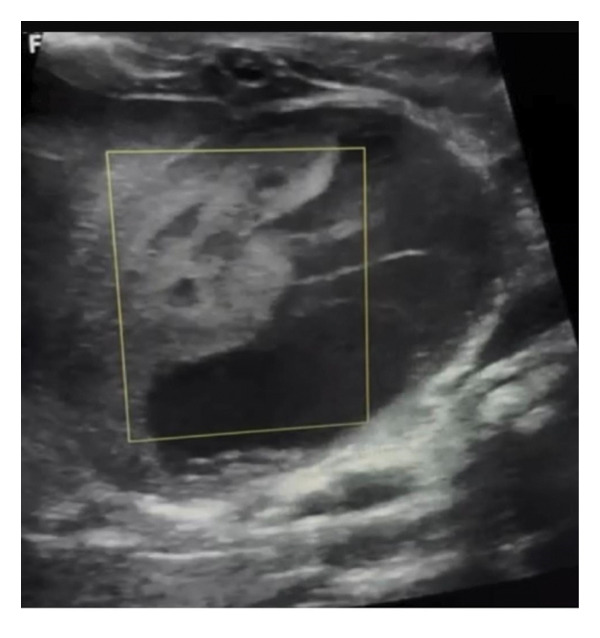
Initial abdominal ultrasound (Day 12) showing a heterogeneous left suprarenal cystic lesion with internal septations, suggestive of adrenal hemorrhage.
